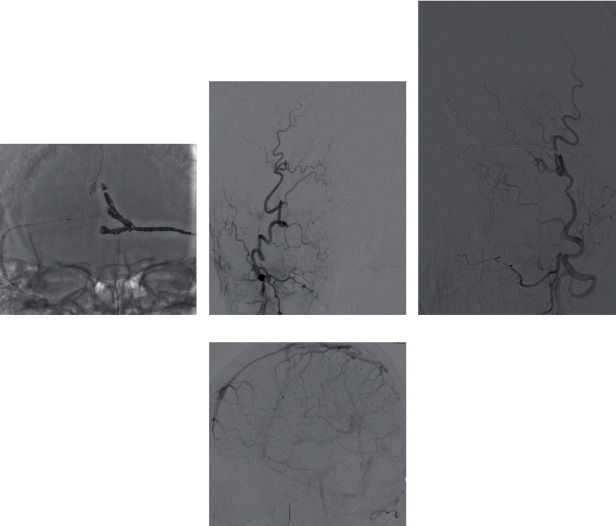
(a–c) The shunt completely disappeared after the embolization from the shunt point near the torcula to the exit of the superior sagittal sinus. ((a) A–P view of X-ray, (b) A-P view of right OAG, and (c) A–P view of left OAG) (d) Venous phase of left internal carotid artery angiography showed a little improvement of venous congestion. OAG: occipital artery angiography.
